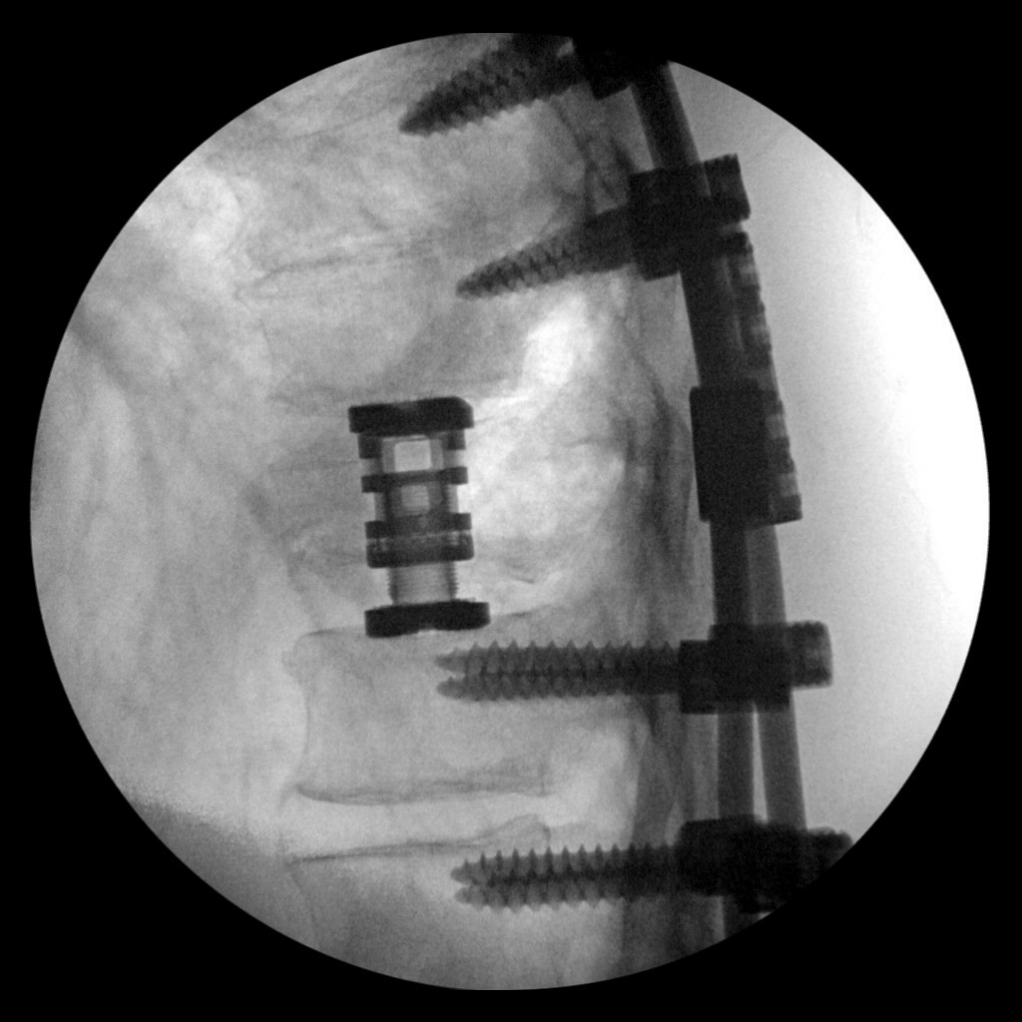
Postoperative lateral radiograph showing placement of T10 cage
